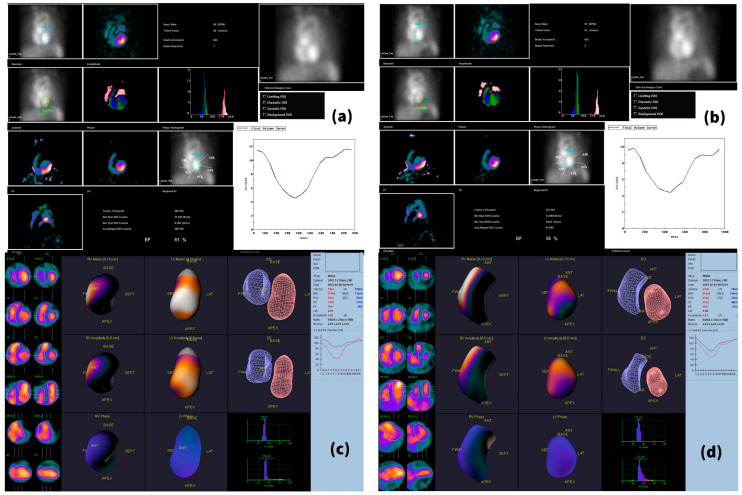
Patient presenting a small decrease in LVEF, not fulfilling the ESC criteria for cardiotoxicity. This 55-year-old female patient suffered from HER2 2+ invasive lobular carcinoma (confirmed through FISH), ER- and PR-negative, previously treated with taxanes. During the 6-month period, she received eight cycles of trastuzumab, pertuzumab and docetaxel. A small decline in LVEF can be observed in both planar and SPECT images, with the EF diminishing from 61% at T0 (a) to 56% at T1 (b) on the planar scans, and from 64% (T0—(c)) to 59% (T1—(d)) on the SPECT acquisitions.
